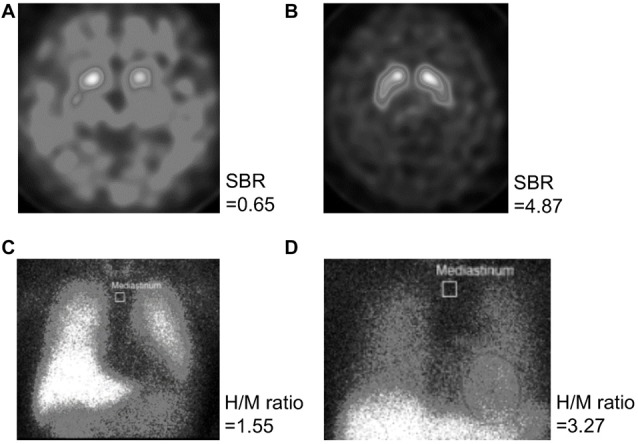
Images of the ^123^I-Ioflupane SPECT and ^123^I-meta-iodobenzylguanidine (MIBG) myocardial scintigraphy. ^123^I-Ioflupane SPECT images of the patients with (A) the lowest and (B) the highest SBR (average of the right and left sides) and ^123^I-MIBG myocardial scintigraphy images of the patients with (C) the lowest and (D) the highest H/M ratio of the early image are shown. Abbreviations: SPECT, single photon emission computed tomography; SBR, specific binding ratio for the striatum; H/M ratio, heart-to-mediastinum ratio.
